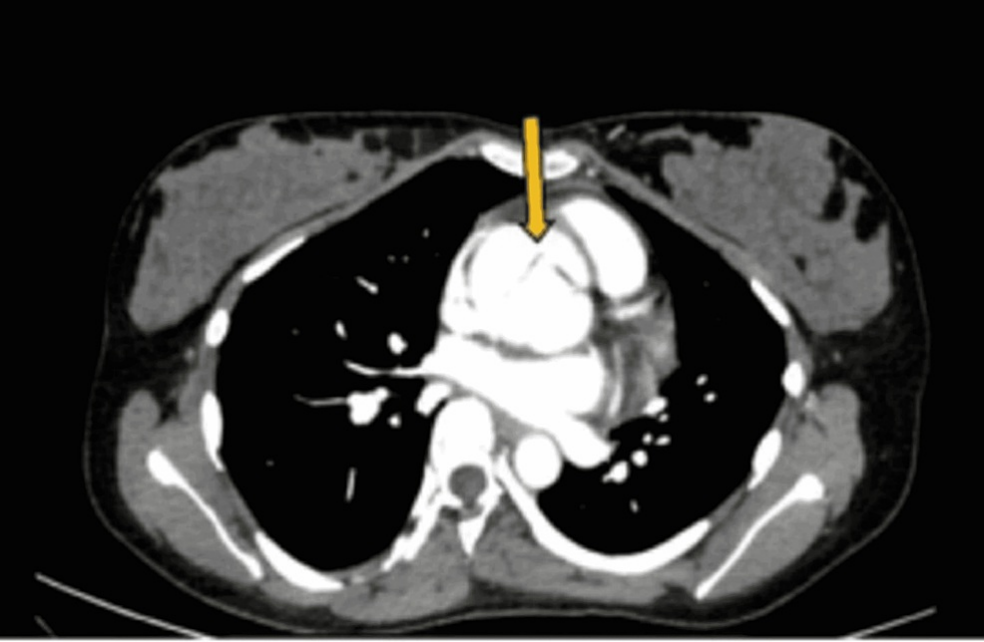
CT aortogram showing Stanford type A aortic dissection (arrow) (axial view)
